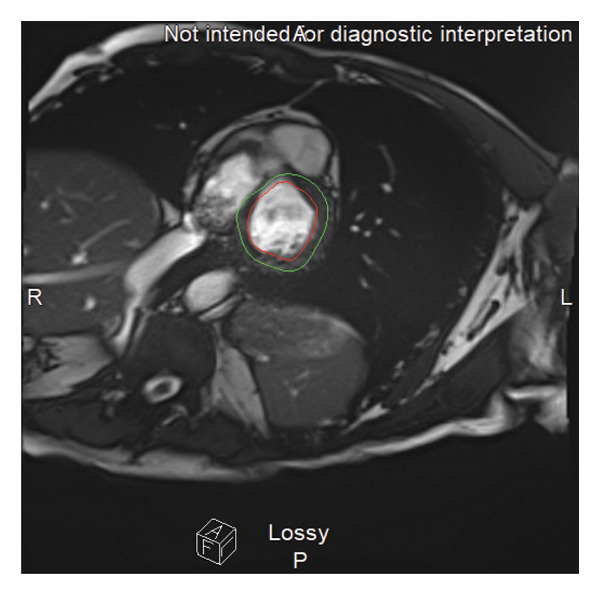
MRI of the heart demonstrating a mobile right atrial mass, intermittently visualized, appearing to arise from the Eustachian valve near the inferior vena cava (red circle). The mass is not attached to any cardiac valves, and there is no associated valvular pathology. These findings are more consistent with thrombus rather than infective vegetation.
